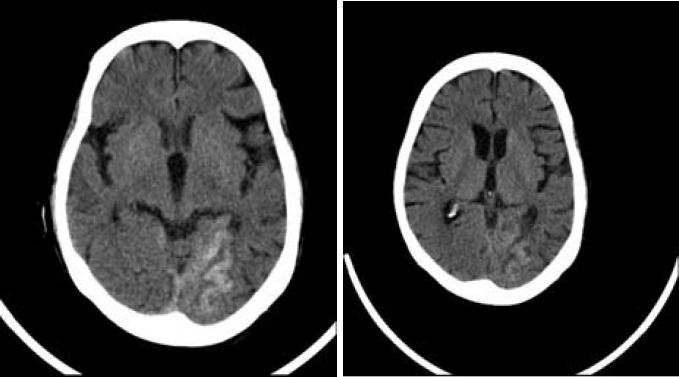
Computerized tomography (CT) scan showing a hemorrhage in the right occipital lobe
